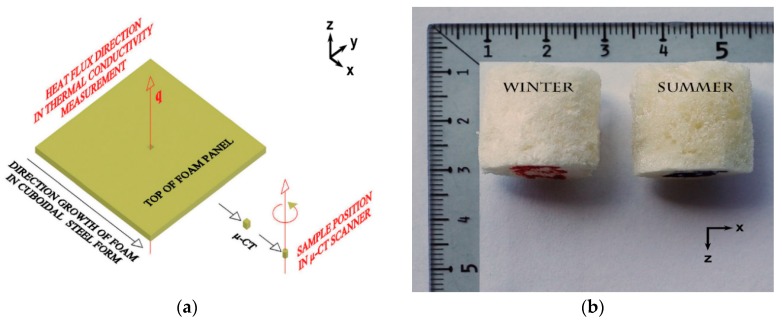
(a) Preparation of the sample for X-ray computed microtomography (µ-CT) measurement from the polyurethane (PU) panel: foam growth along the x-axis, cutting a cuboidal, and further forming the cylindrical sample. The sample rotation in the µ-CT scanner is shown around the z-axis—parallel to vector q and perpendicular to the scanner holder in xy-plane. In this figure, the sample to panel size ratio is kept the same as the original; (b) The enlarged WINTER (W)/SUMMER (S) foam samples are shown over the xz-plane.
